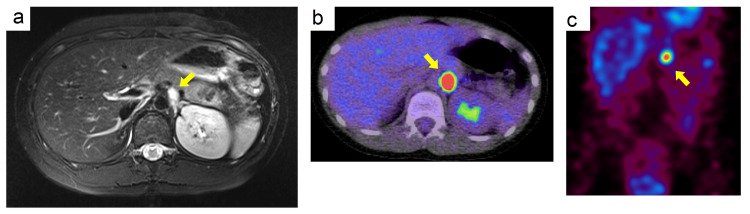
Imaging studies of the patient at recurrence Axial abdominal MRI T2-weighted images (a), 18F-FDG-PET (b), and coronal 123I-MIBG scintigraphy (c). Tumors are indicated by yellow arrows. FDG: F-18 fluorodeoxyglucose; PET: positron emission tomography; MIBG: meta-iodobenzylguanidine
